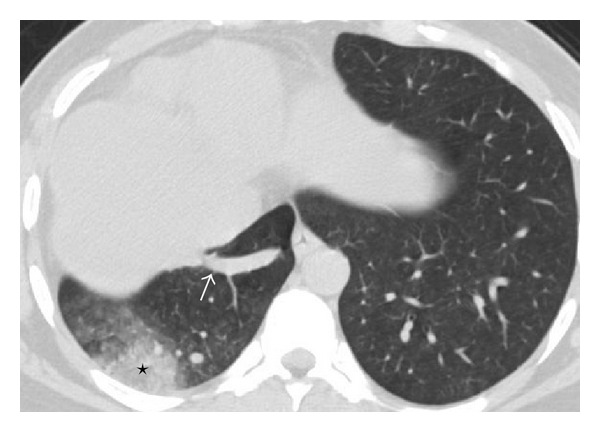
CT axial image depicts sequestration as patchy consolidation at right posterobasal region (asterix), meandering right inferior pulmonary vein (arrow).
